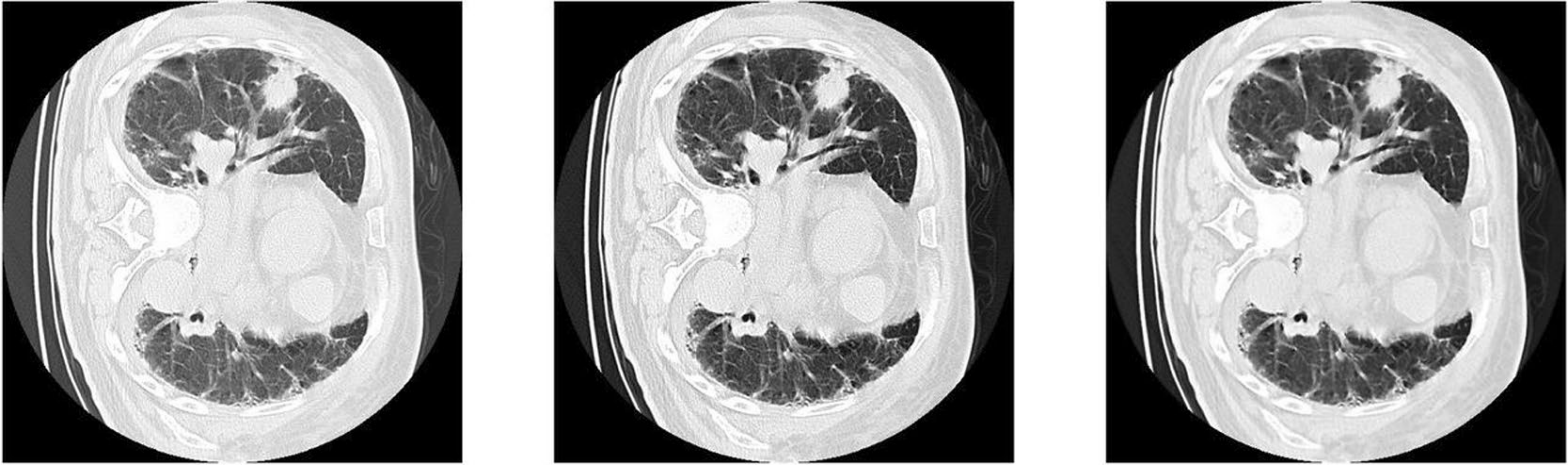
From left to right, Original CT image, With CLAHE, With CLAHE and Wiener.
